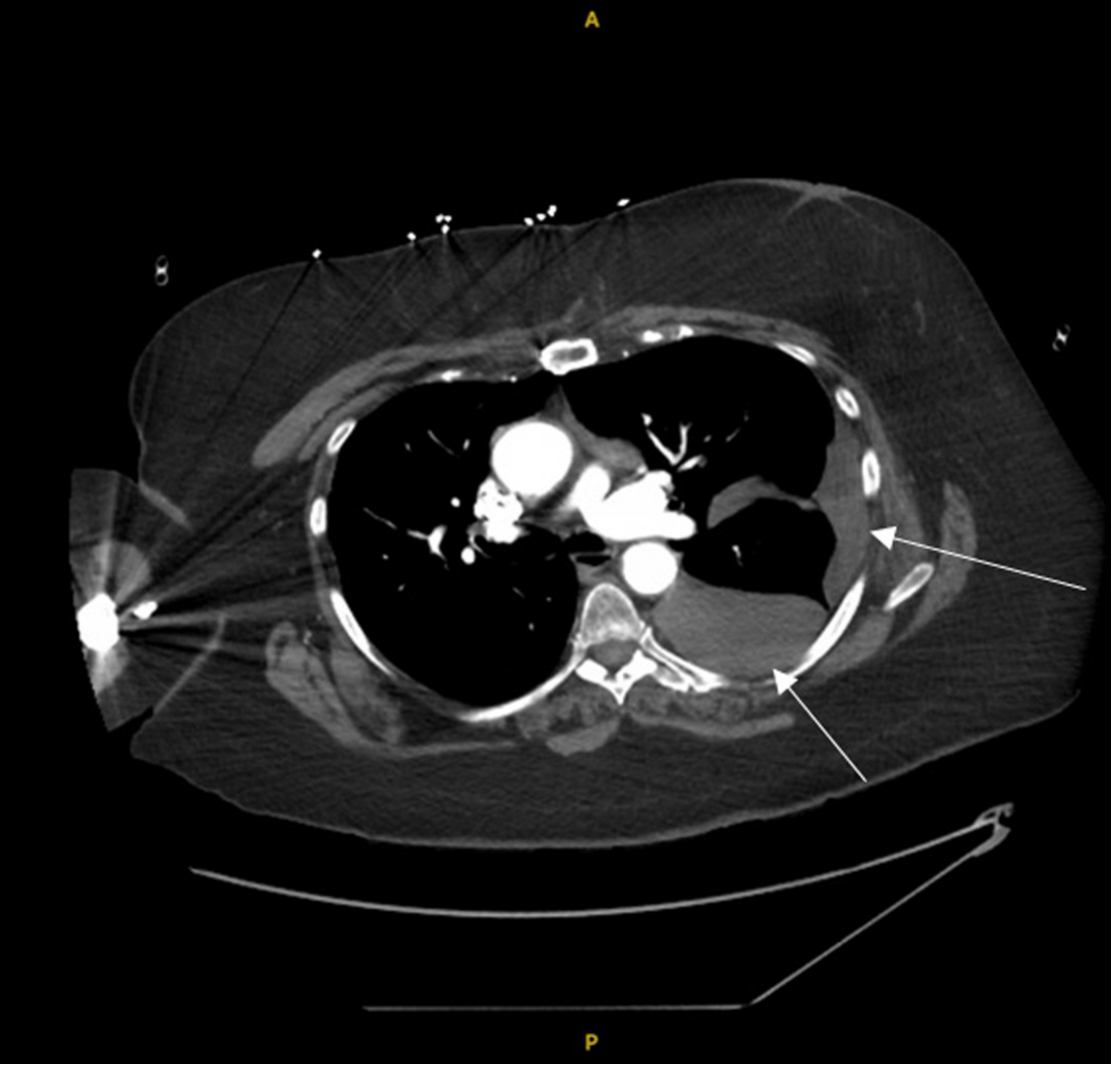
Computed tomography angiography of the chest; axial image showing dense left pleural effusion (arrows).
